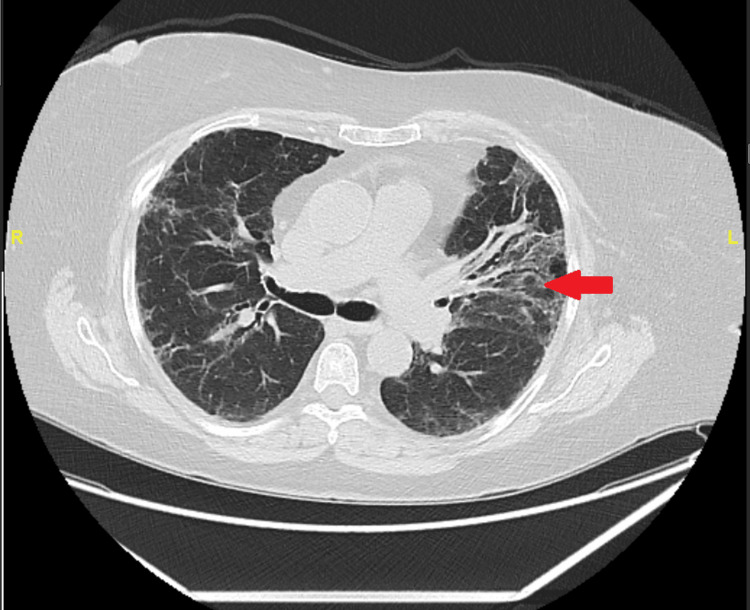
Contrast-enhanced CT of the chest showing fibrotic changes with architectural distortion and traction bronchiectasis, without new cavitary lesions or evidence of active pulmonary vasculitis. CT: computed tomography
